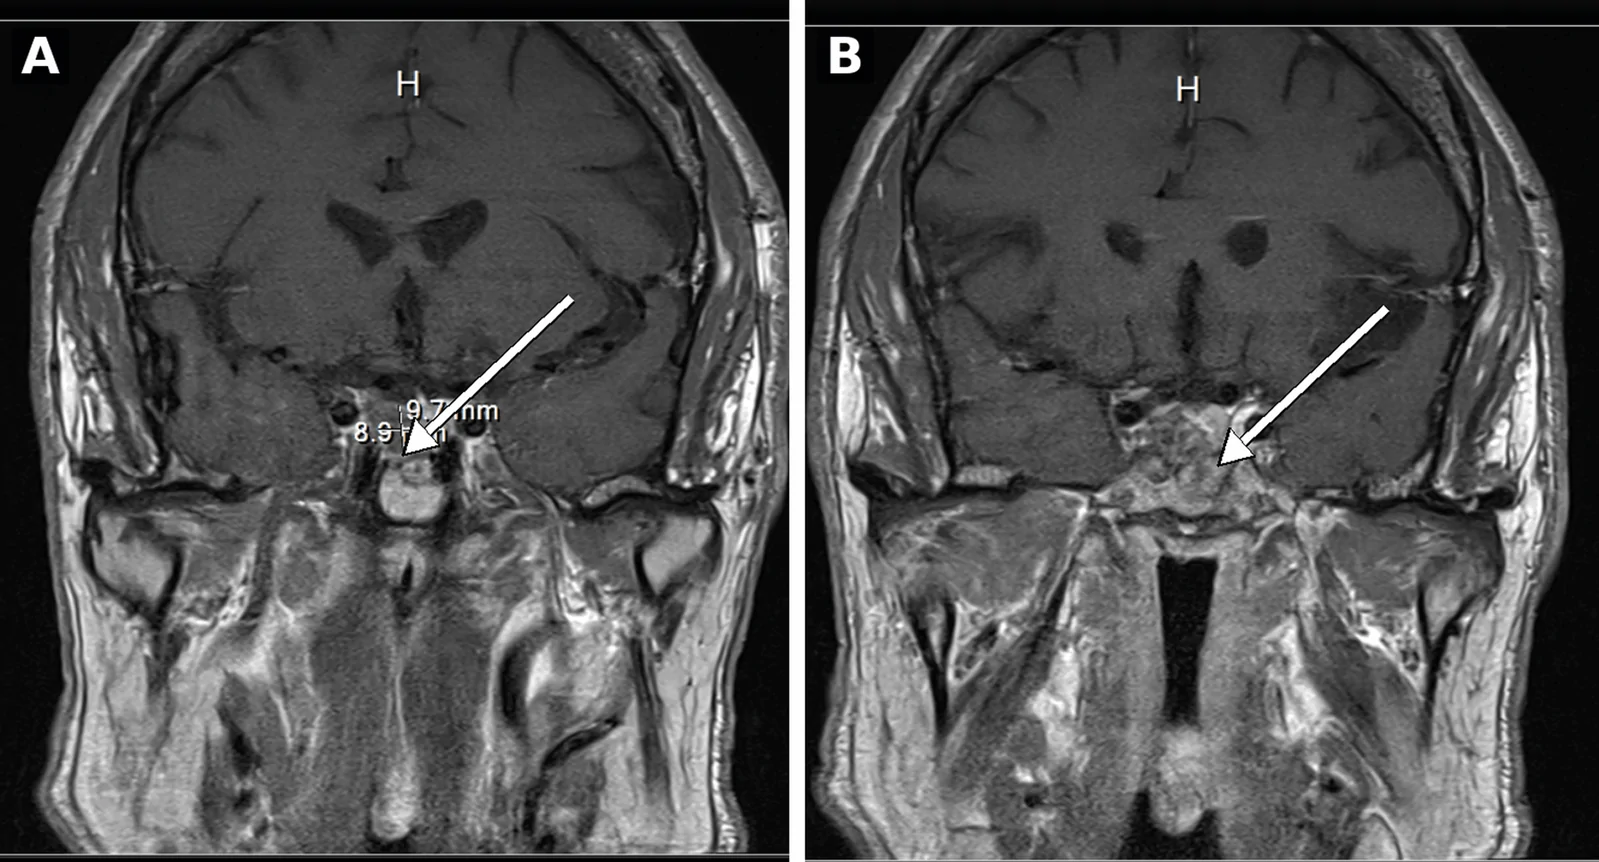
Surveillance Pituitary MRI at Six Months Confirming Durable Resection Surveillance pituitary MRI obtained in September 2025, approximately six months after surgery, confirming durable resection of the macroadenoma. (A) Coronal T1-weighted post-contrast image showing the residual surgical cavity (arrow) measuring approximately 9.7 × 8.9 mm without a gross residual tumor. (B) Coronal T1-weighted post-contrast image at a slightly different level showing no residual enhancing tumor.
